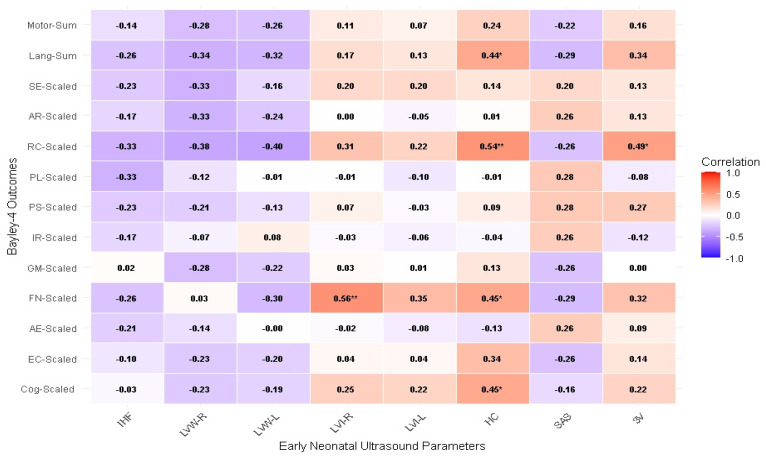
Spearman correlations between early neonatal ultrasound parameters and Bayley-4 outcomes. * Asterisks indicate statistical significance (* *p* < 0.05;  *p* < 0.01).
